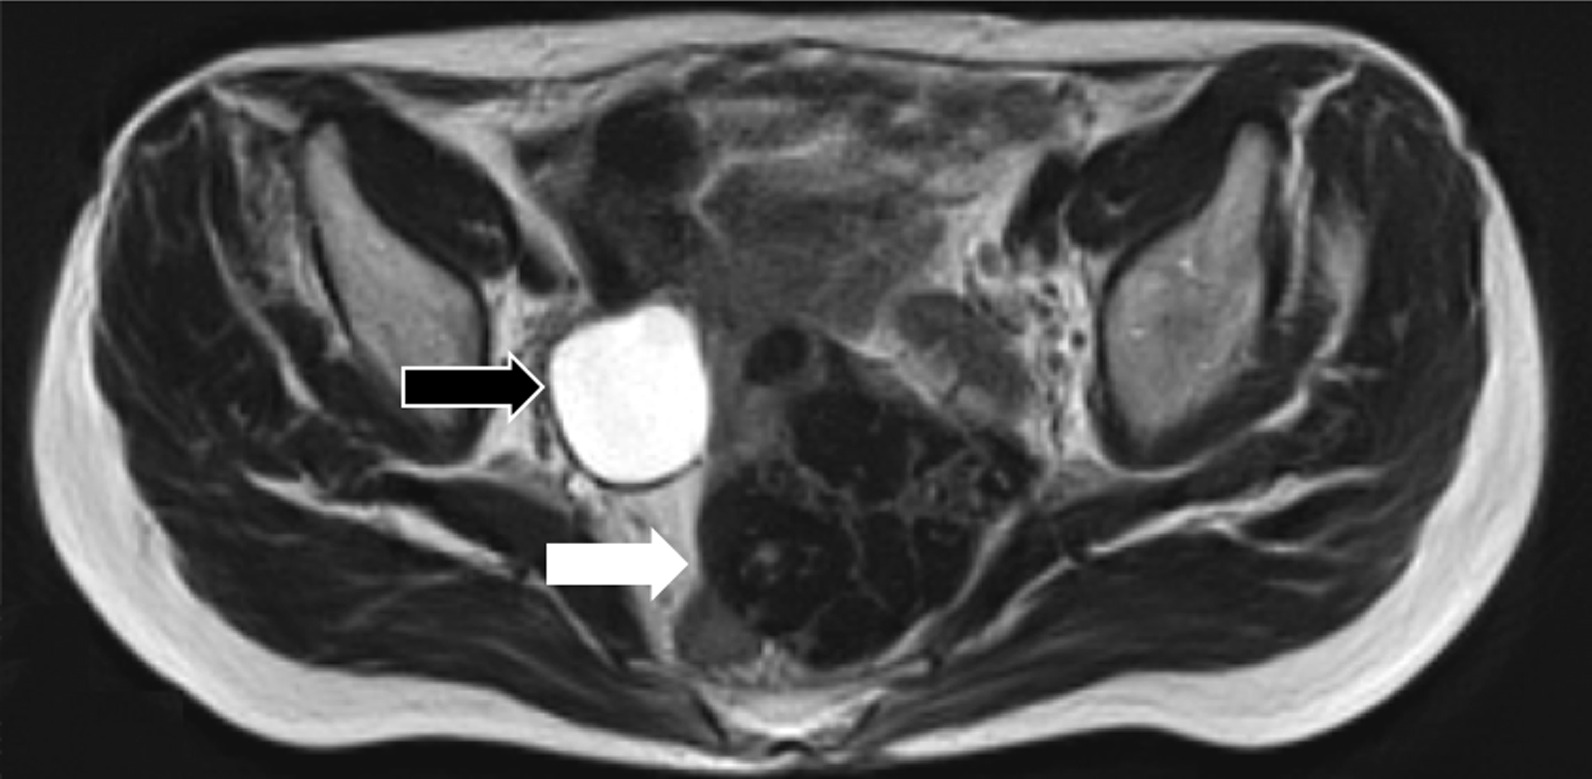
Magnetic resonance imaging of the pelvis. T2-weighted image reveals an 8 cm low-density tumor (white arrow) located at the posterior surface of the uterus and a 5 cm high-density simple cyst (black arrow) located at the right para-ovary and without solid compartment
